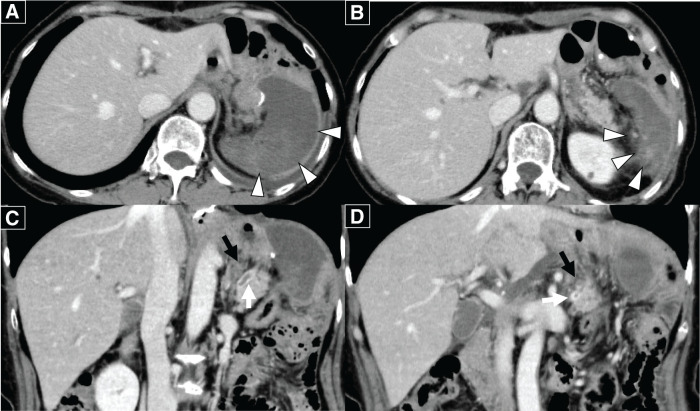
(A, B) Contrast-enhanced abdominal CT scan showed poor perfusion throughout the splenic parenchyma. Slight contrast enhancement in the splenic parenchyma remains (white arrowheads). (C, D) A thrombus was noted in the splenic vein (white arrow) and in the tortuous segment of the splenic artery near the pancreatic body (black arrow).
